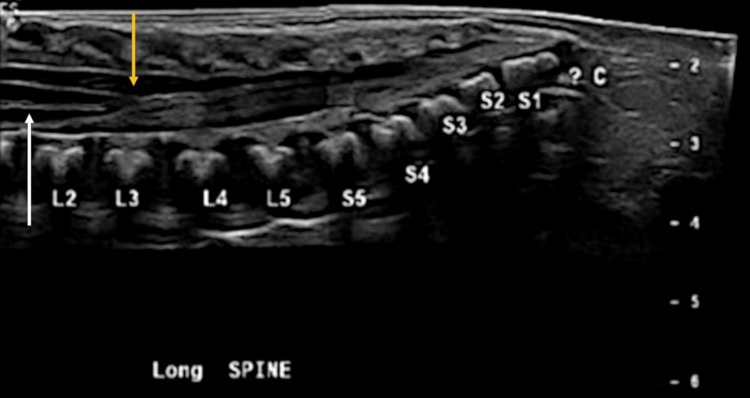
Spinal ultrasound Longitudinal spinal ultrasound demonstrates a low-lying cord with the tip of the conus medullaris non-dependently positioned at the middle of the L3 vertebral level (yellow arrow). Incidental finding of a split central echogenic complex likely represents transient dilatation of the central canal (i.e., ventriculus terminalis) (white arrow). Radiological images obtained by the authors.
